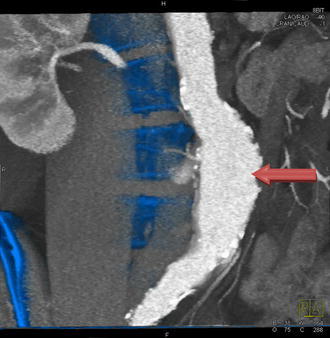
CT angiography of AAA. *Arrow* the site of sample collection from the AAA wall
